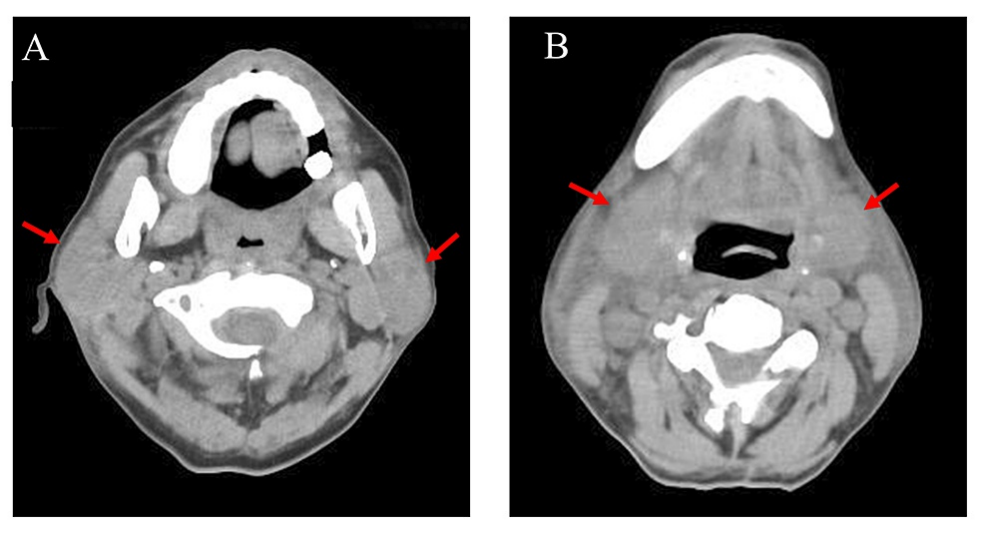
Plain computed tomography scan of the neck on day six post-chimeric antigen receptor T-cell (CAR-T) cell infusion in Case 1. A. Swelling of bilateral parotid glands (red arrows). B. Swelling of submaxillary glands (red arrows).
